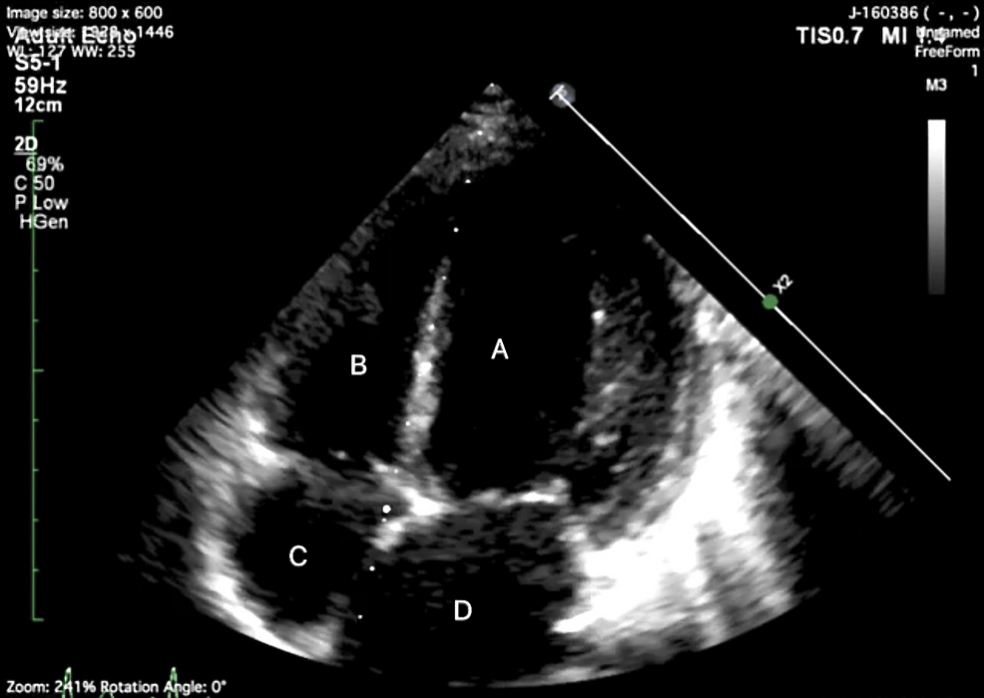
Apical four-chamber view showing left ventricle and left atrium dilatation A: left ventricle; B: right ventricle; C: right atrium and D: left atrium
